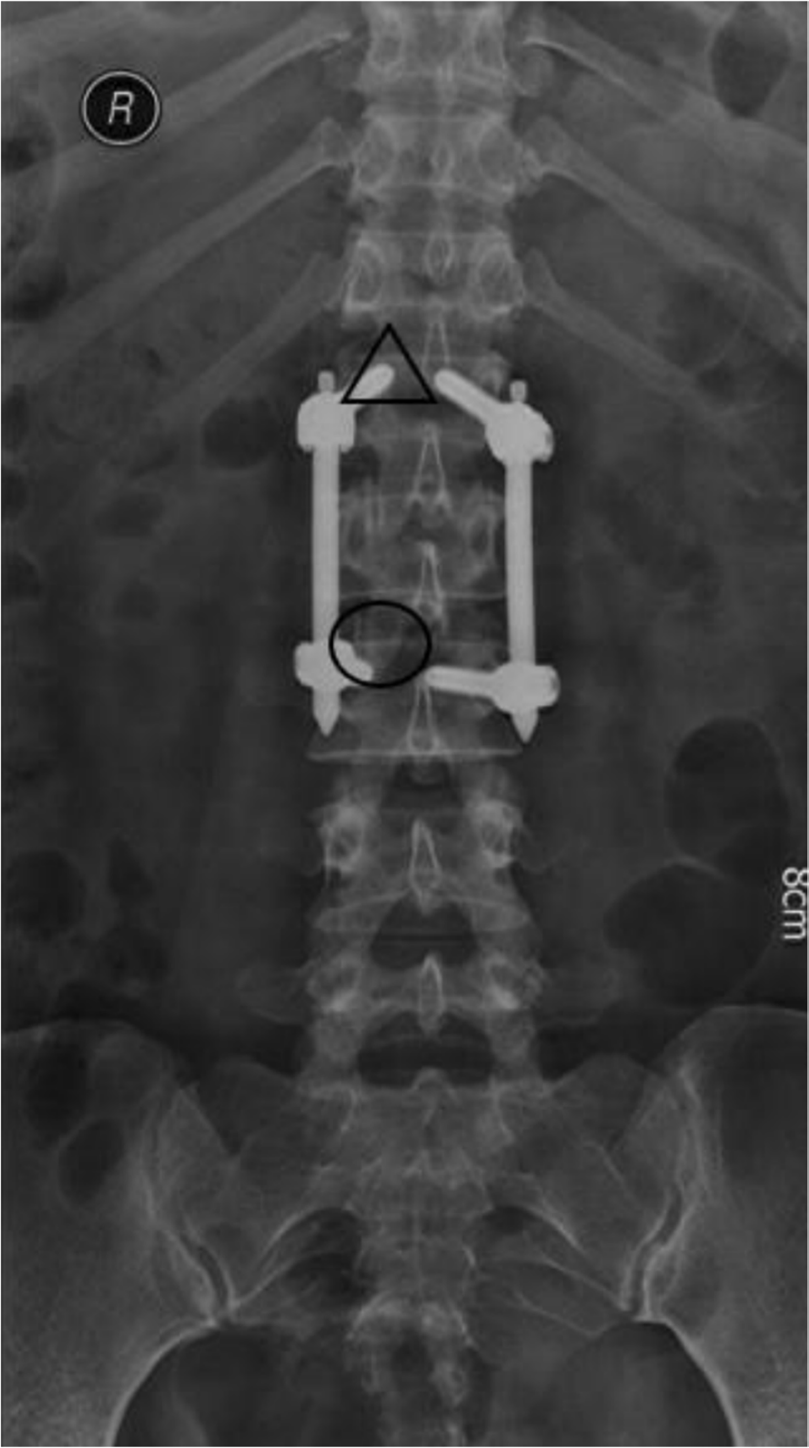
X-ray view of the cranial and caudal facet joints for each patient in the study. ○ shows the caudal facet joints, and △ shows the cranial facet joints
